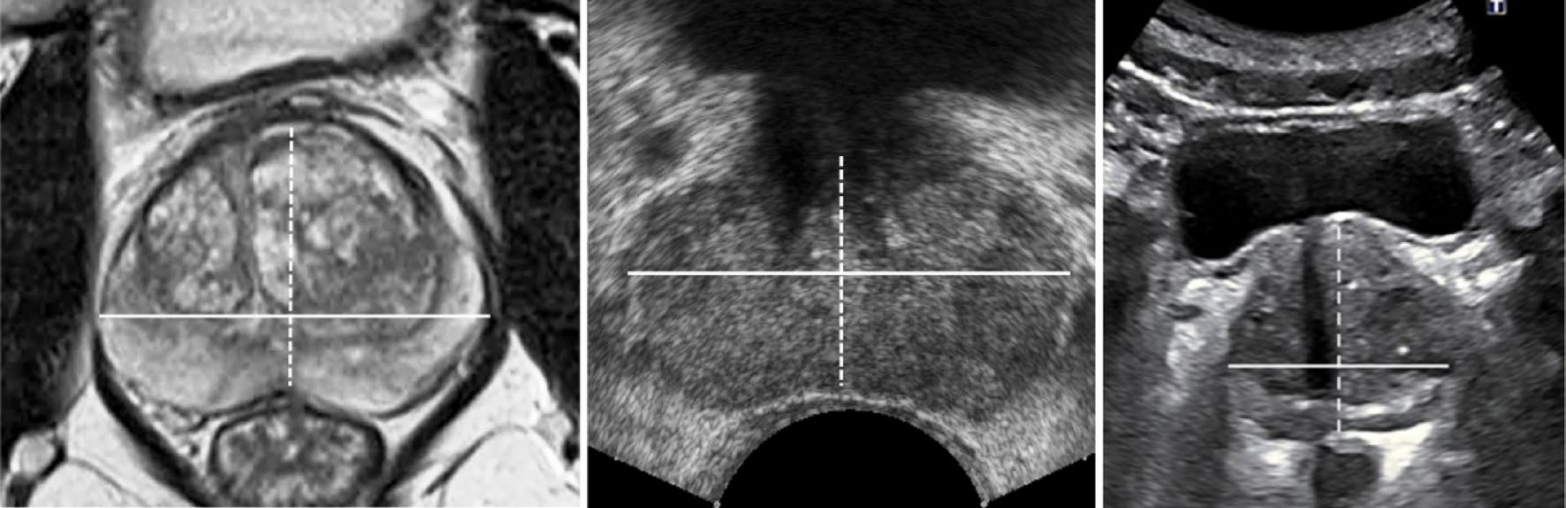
Comparison of different prostate imaging techniques: MRI—Left; TRUS^21^—Middle; and AUS^19^—Right. The major dimensions of the prostate are indicated.
